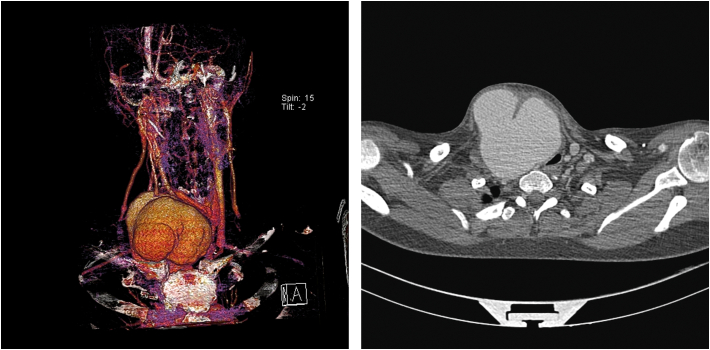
Preoperative contrast-enhanced computed tomography angiogram (CTA): the pseudoaneurysm of the innominate artery causing pressure effect on the trachea.
